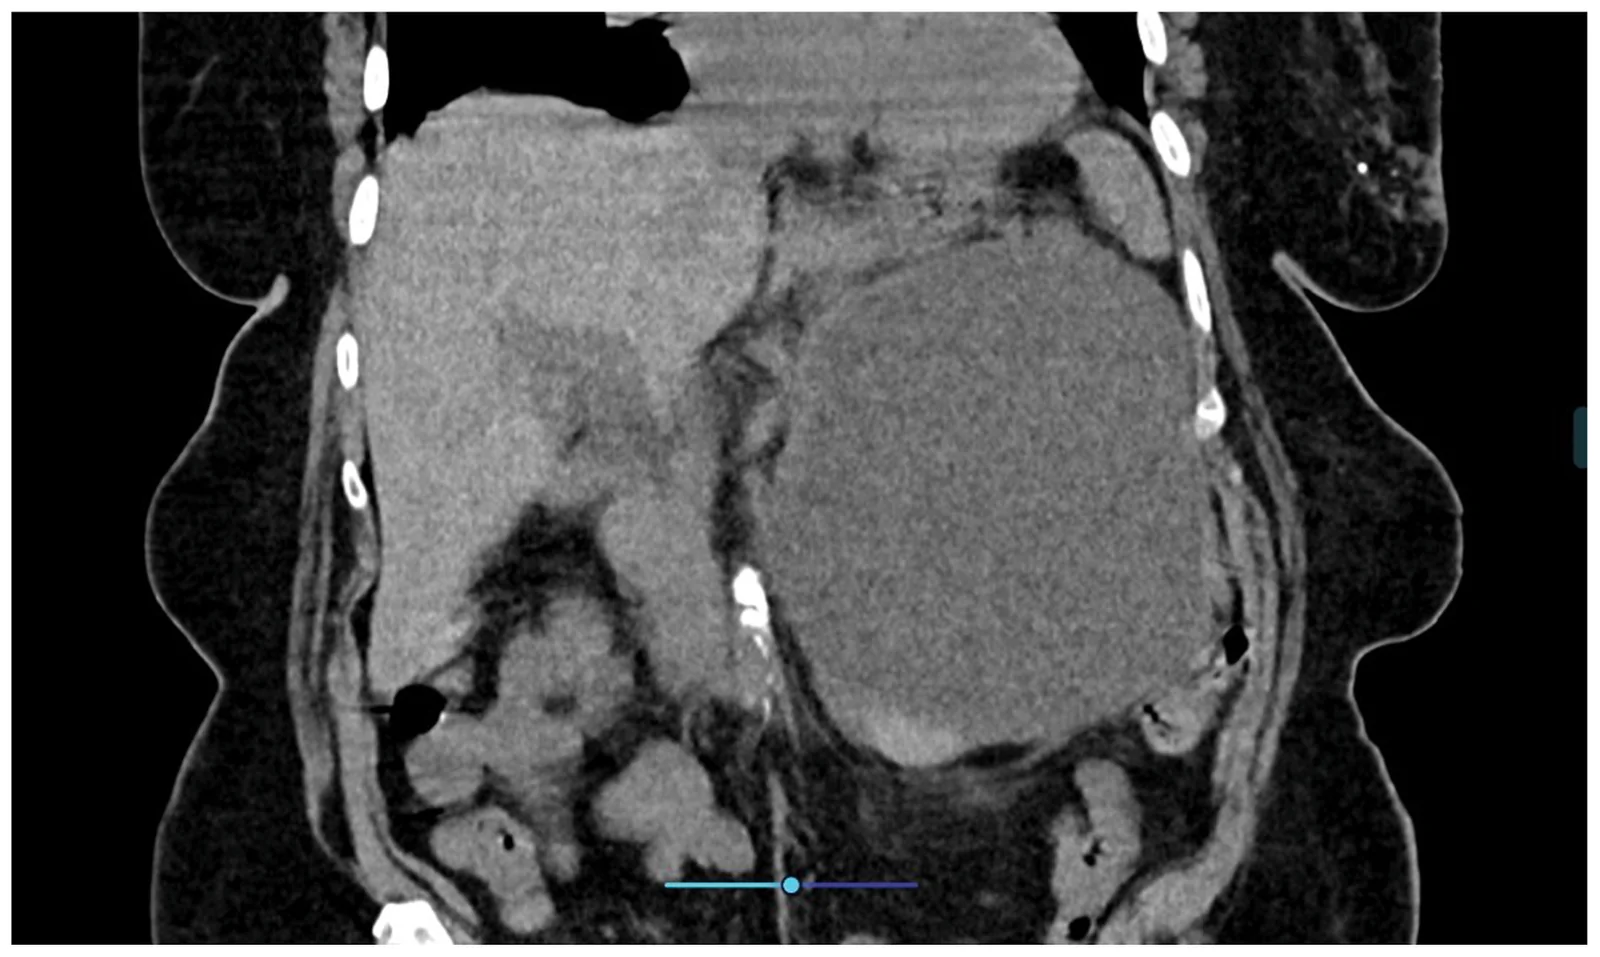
Complex left renal cystic mass measuring up to 17.9 × 12.6 × 16.6 cm, previously 17.2 × 12.6 × 15.5 cm. A hyperdense component is again noted along the posterior aspect of the lesion, corresponding to T1-hyperintense products on previous magnetic resonance imaging (MRI). No new solid component is identified, although evaluation is limited by the absence of intravenous contrast. Right renal cysts are again visualized, the largest measuring up to 3.0 cm, previously 3.4 cm. There is no noncontrast evidence of a suspicious right renal mass, renal calculus, or hydronephrosis.
